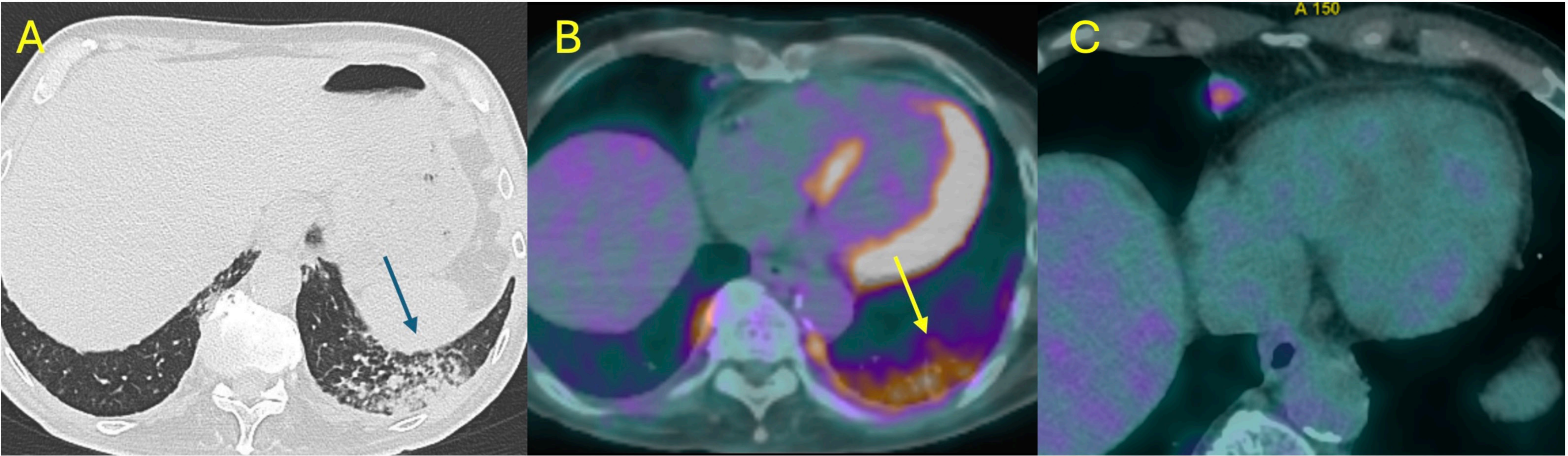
(A) CT scan demonstrated lung consolidation in the lower left lobe (blue arrow). (B) PET scan showed an intense captation in the lower left lobe (yellow arrow). (C) PET scan revealed a significant captation in a right diaphragmatic lymph node.
